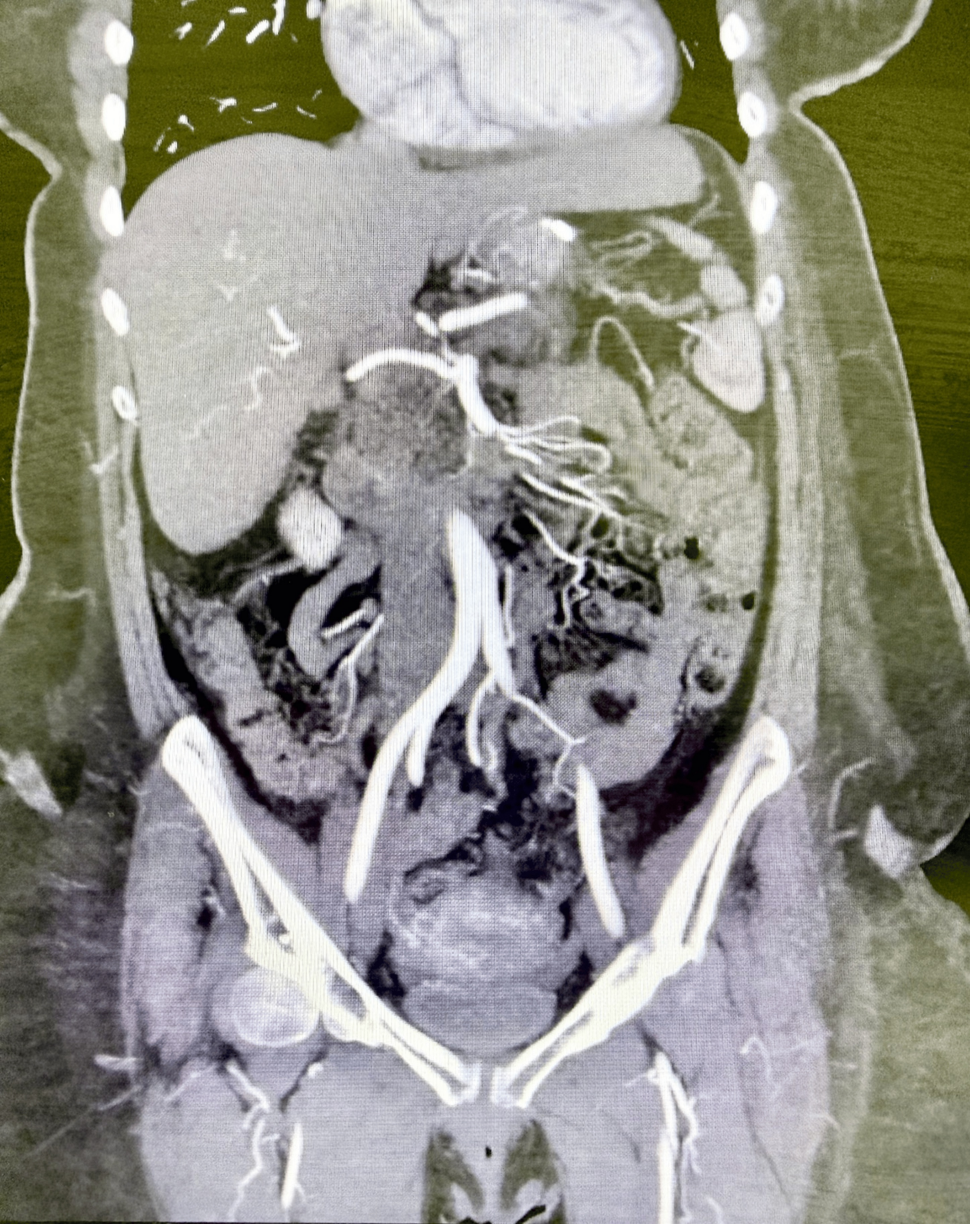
Contrast-enhanced CT abdomen demonstrating venous thrombosis with pancreatic involvement. Coronal contrast-enhanced CT image demonstrating thrombosis of the mesenteric venous system with surrounding mesenteric congestion and inflammatory changes involving the pancreas. CT: computed tomography.
